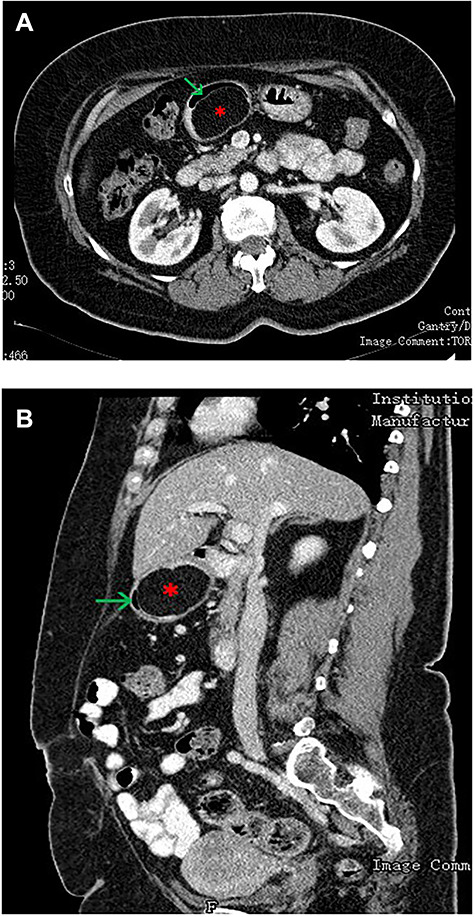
(A—coronal plane; B—sagittal plane): CT scan of the abdomen showing a large well-encapsulated and fat-attenuated submucosal lesion (8 × 3 cm) in the posterior wall of the gastric antrum, which causes lumen obstruction (^*^gastric lipoma, arrow—gastric lumen).
